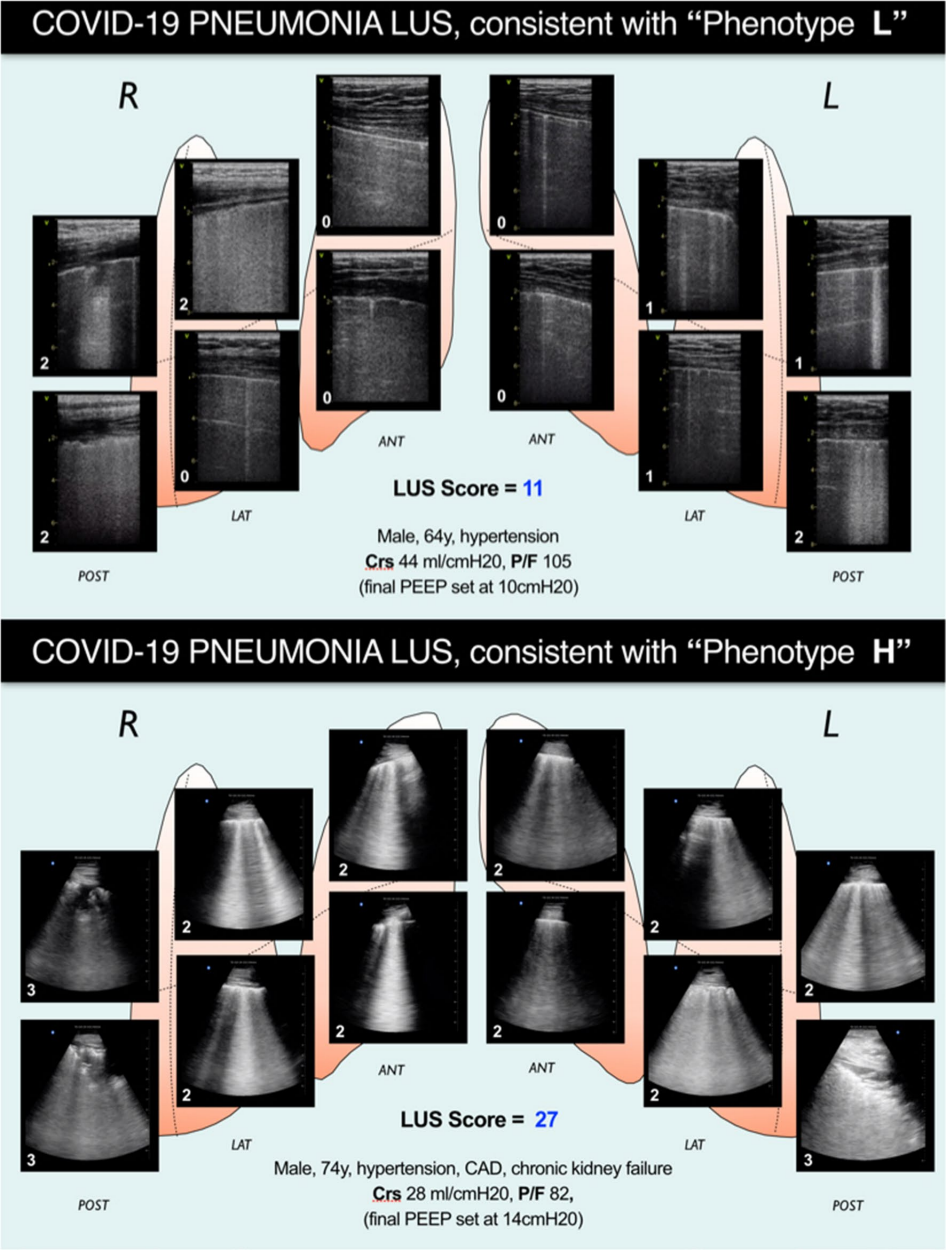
Examples of lung ultrasound cumulative patterns of patients presenting with a similar degree of hypoxemia, but very different degree of aeration and respiratory mechanics characteristics, and recalling the recently proposed COVID-19 pneumonia phenotypes [89]. Patient on upper panel presents a nearly normal respiratory system compliance and LUS evidence of a milder lung involvement, reflected in a total LUS score of 11. This suggests a lung condition matching which has been recently described as “Phenotype L,” based on CT findings, and characterized by low lung elastance and low ventilation/perfusion ratio (explaining the severe hypoxia). Based on this imaging and on respiratory mechanics findings, final PEEP was set at 10 cm H_2_0. Upper panel shows LUS evidence of a more diffuse and severe diffuse sonographic interstitial syndrome (cause of the shunt and the severe hypoxia), yielding a total LUS score of 27. Respiratory mechanics characteristics recall what has been described as “Phenotype H” (COVID-19 pneumonia: high lung elastance, high right-to-left shunt). Based on this imaging and on respiratory mechanics findings, PEEP was set at 14 cm H_2_0 after a stepwise recruiting maneuver. LUS, lung ultrasound
